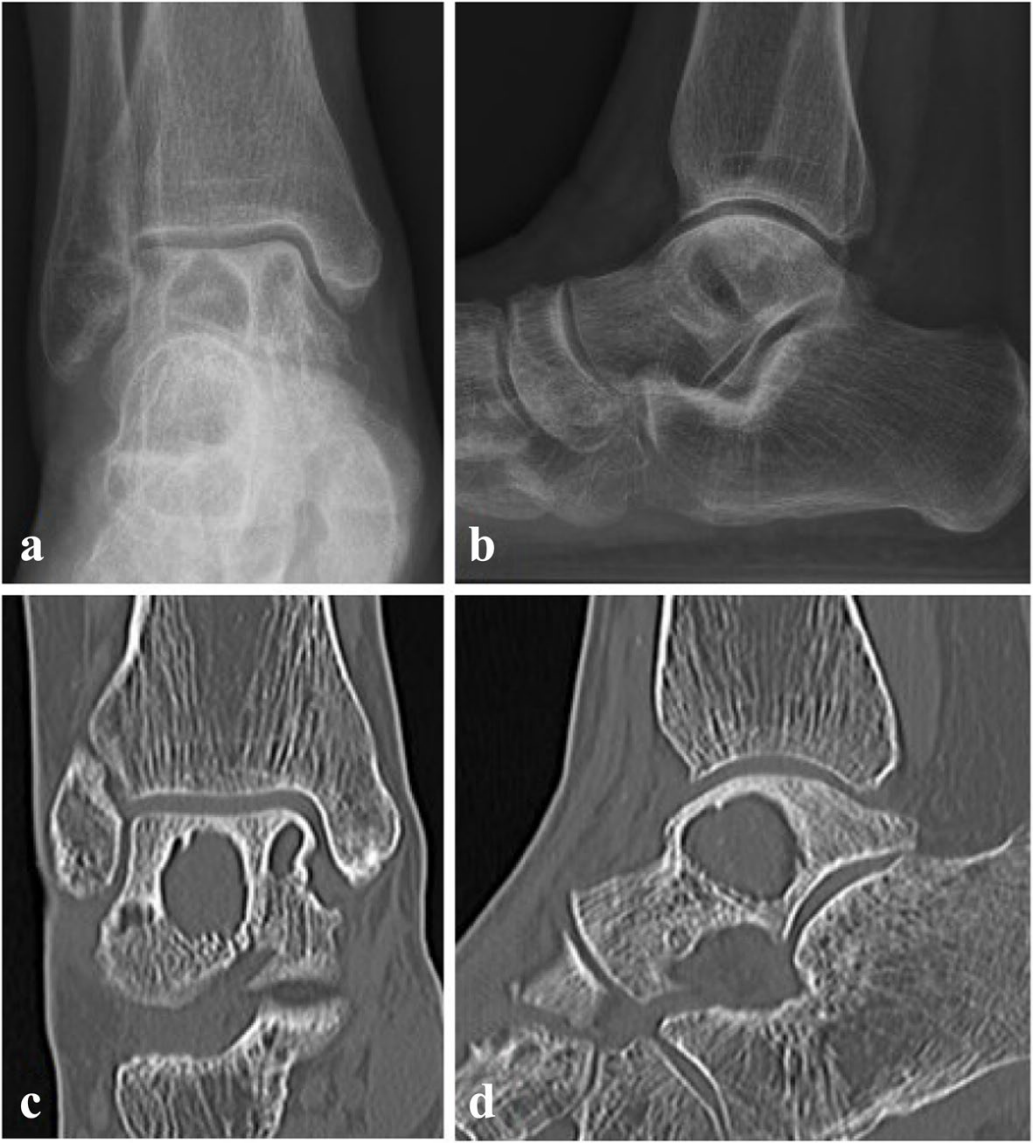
Plain radiographs and CT images of postoperative 16 months. Plain radiographs (a, b) and CT (c, d) showing slightly thickened cystic wall without any evidence of talar collapse
